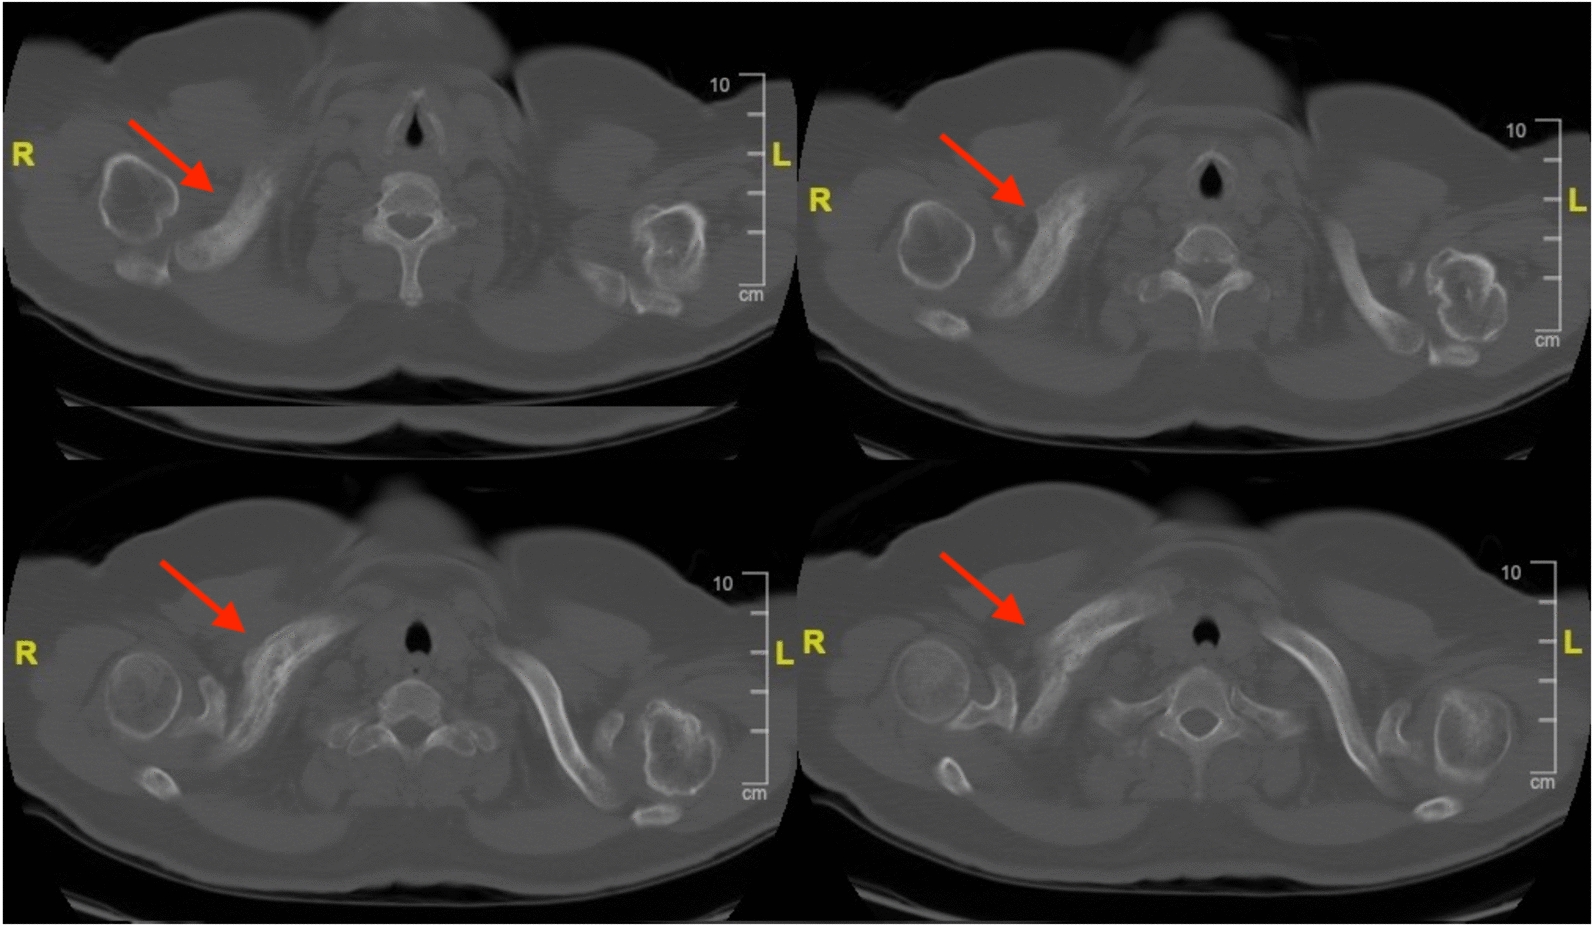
CT of chest in bone window disclosed heterogenous bone density with fracture and adjacent soft tissue lesion of right clavicle (Arrows: clavicle lesion)
